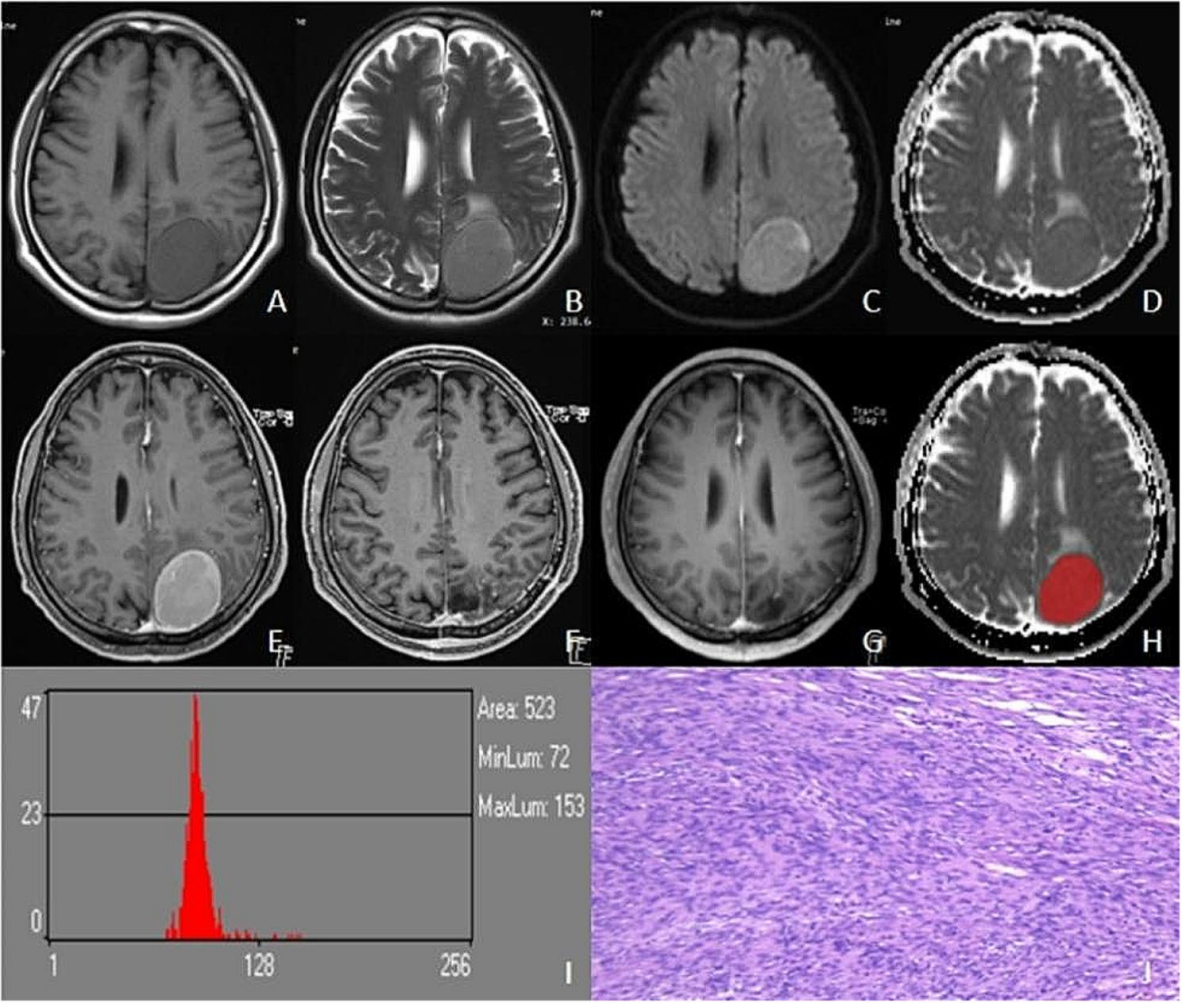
Male, 52 years old, left parieto-occipital fibroblastic meningioma with no recurrence till 28 months. The left parieto-occipital region showed a round iso T1WI (A) and slightly longer T2WI signal (B), with signal homogeneity, slightly hyperintensity on DWI (C), and iso-signal on ADC (D). T1C showed significant homogeneous enhancement (E), and there was no recurrence at 3-month (F) and 28-month postoperative review (G). Outlined ROI (H), ADC histogram parameters values were as follows: mean, 94.072; variance, 320.36; skewness, 4.7907; kurtosis, 30.683; ADCp1, 75; ADCp10, 83; ADCp50, 91; ADCp90, 103; ADCp99,184 (I). Pathology (HE×100): fibroblastic meningioma KI-67% was 2% (J)
